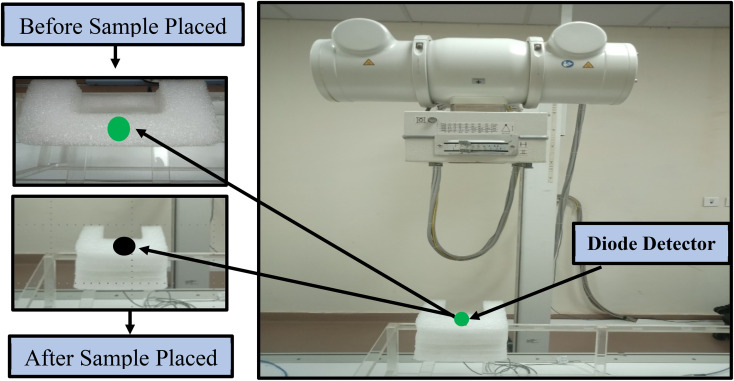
Experimental setup for irradiating 60 keV, 80 keV, and 100 keV of X-ray energies.
